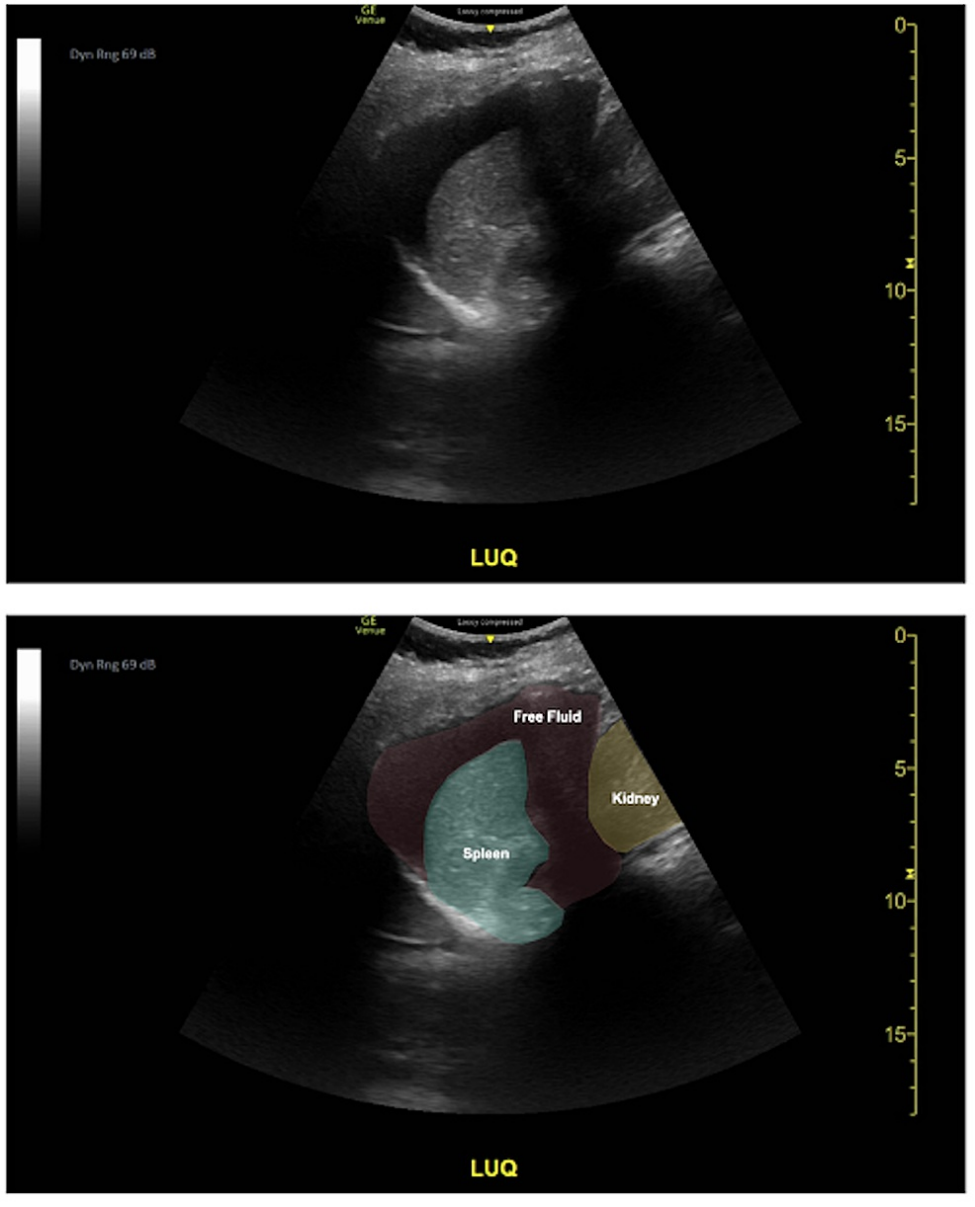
Left upper quadrant view seen on bedside ultrasonography. To obtain this view, the transducer is placed on the posterior axillary line at approximately the 8th rib as the spleen is more posterior and superior in relation to the liver. This image is similar to that of the RUQ and demonstrates the diaphragm, spleen, kidney. In this image we appreciate an anechoic (black) area between the spleen and kidney as well as superiorly to the spleen. The lower image is a color-coded version of the original image for visual interpretation.
